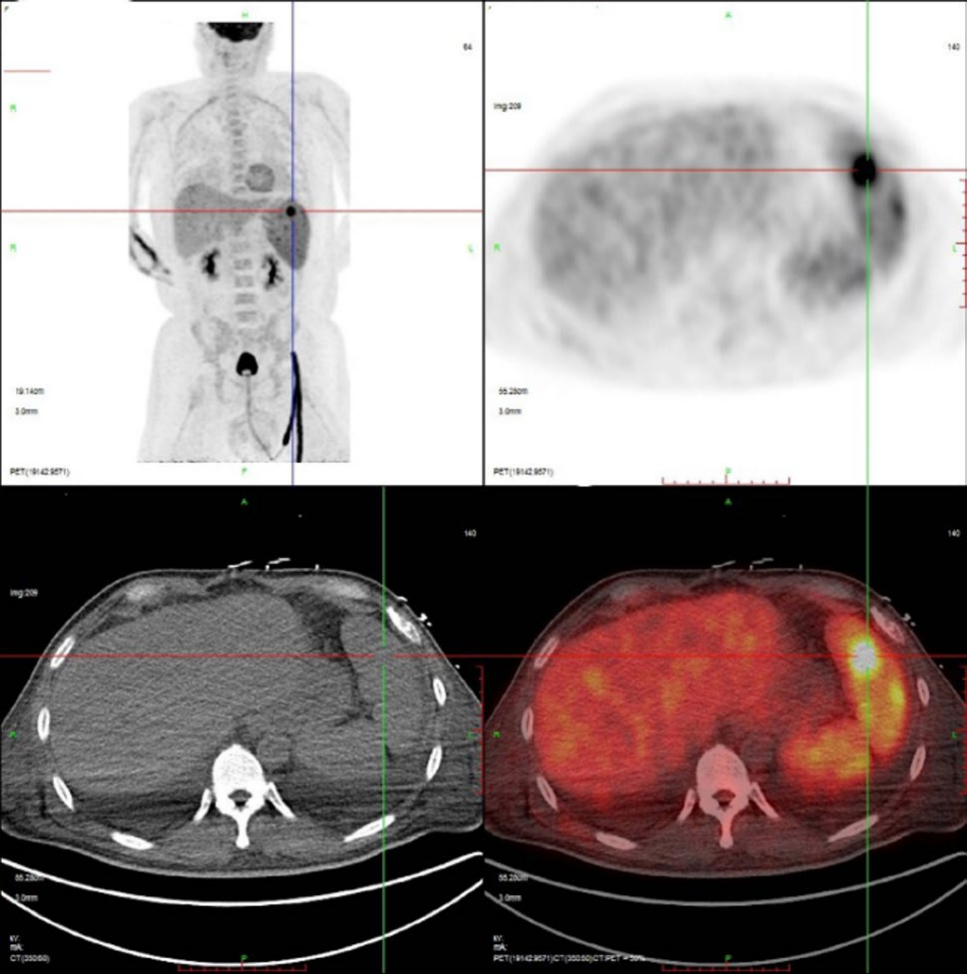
PET-CT imaging demonstrates splenomegaly with focal heterogeneous FDG uptake (SUVmax 9.0) in an area measuring approximately 3.0 × 2.0 cm. The elevated SUVmax indicates active lymphomatous infiltration and confirms extranodal involvement
